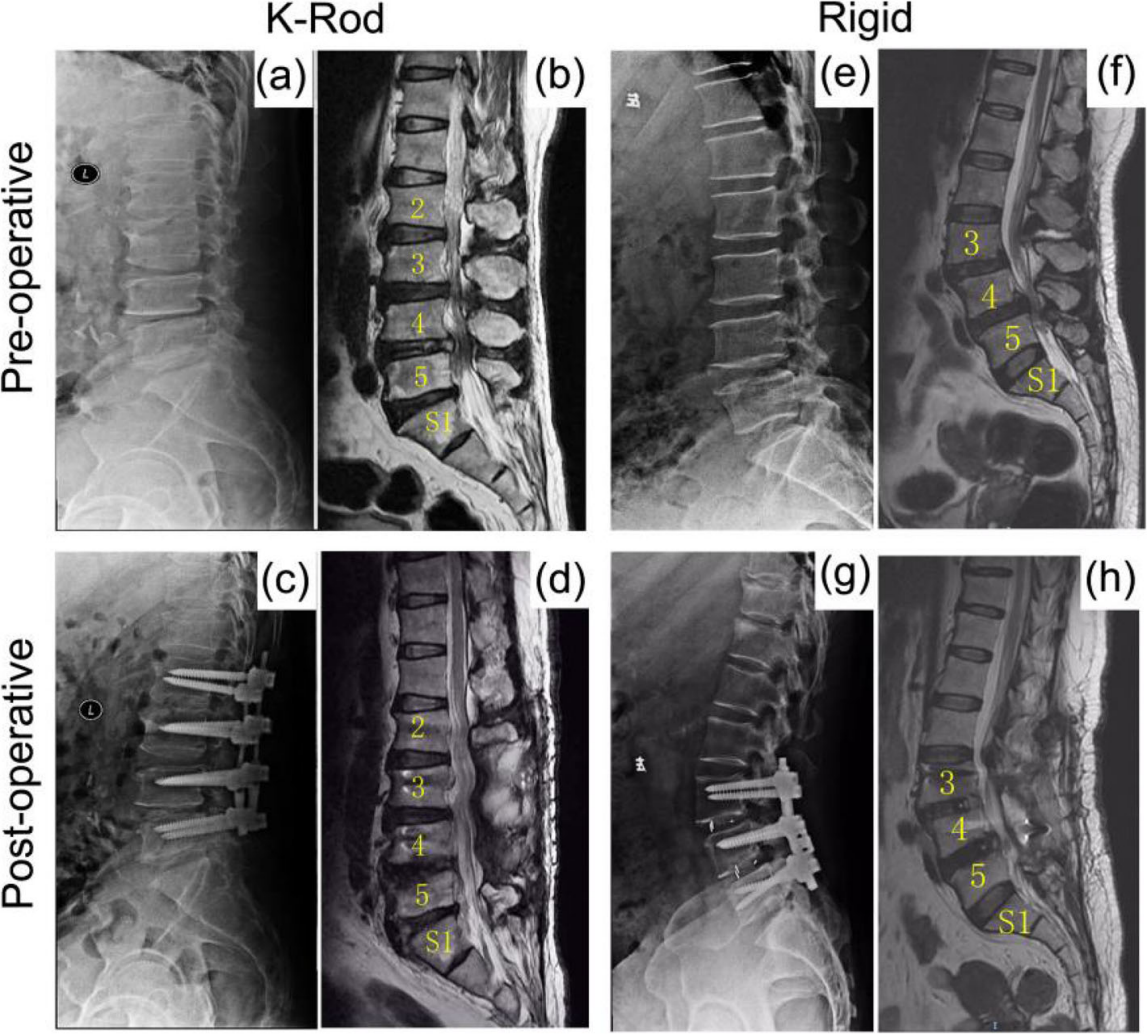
Comparison of pre-operative and post-operative imaging: a-d: K-rod; e-g rigid (DR: a, c, e and g; MRI: b, d, f & h); a, b, e and f: pre-operative images; c, d, g & h: post-operative images of patients
